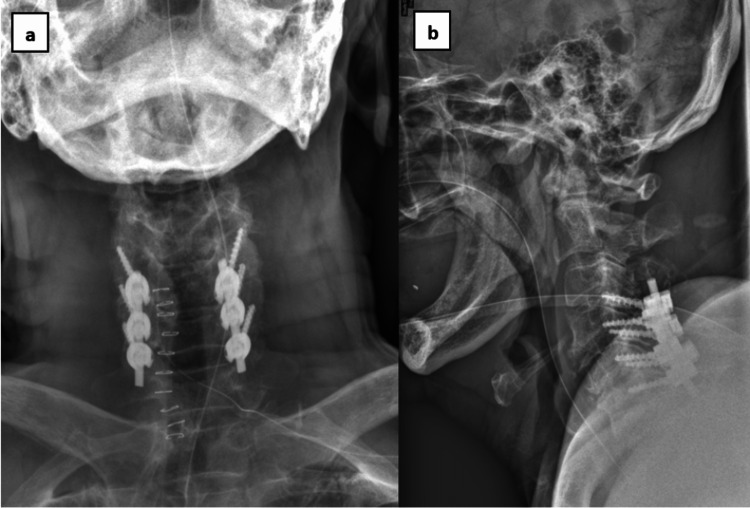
Post-operative cervical radiographs reveal the accurate trajectory of lateral mass screws, along with the restoration of cervical lordosis and disc height. a) anteroposterior view, b) lateral view
